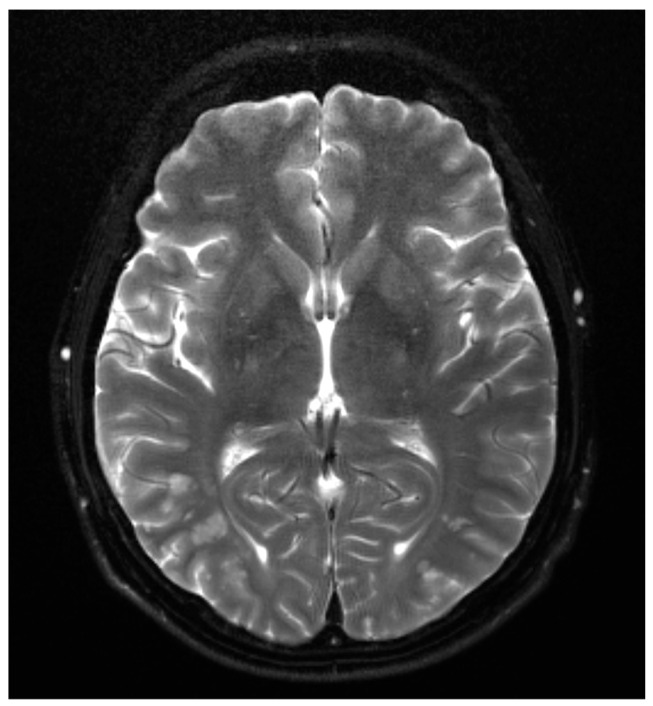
Brain MRI: Brain MRI showing numerous small bilateral cerebral and cerebellar infarcts predominantly involving watershed territories.
